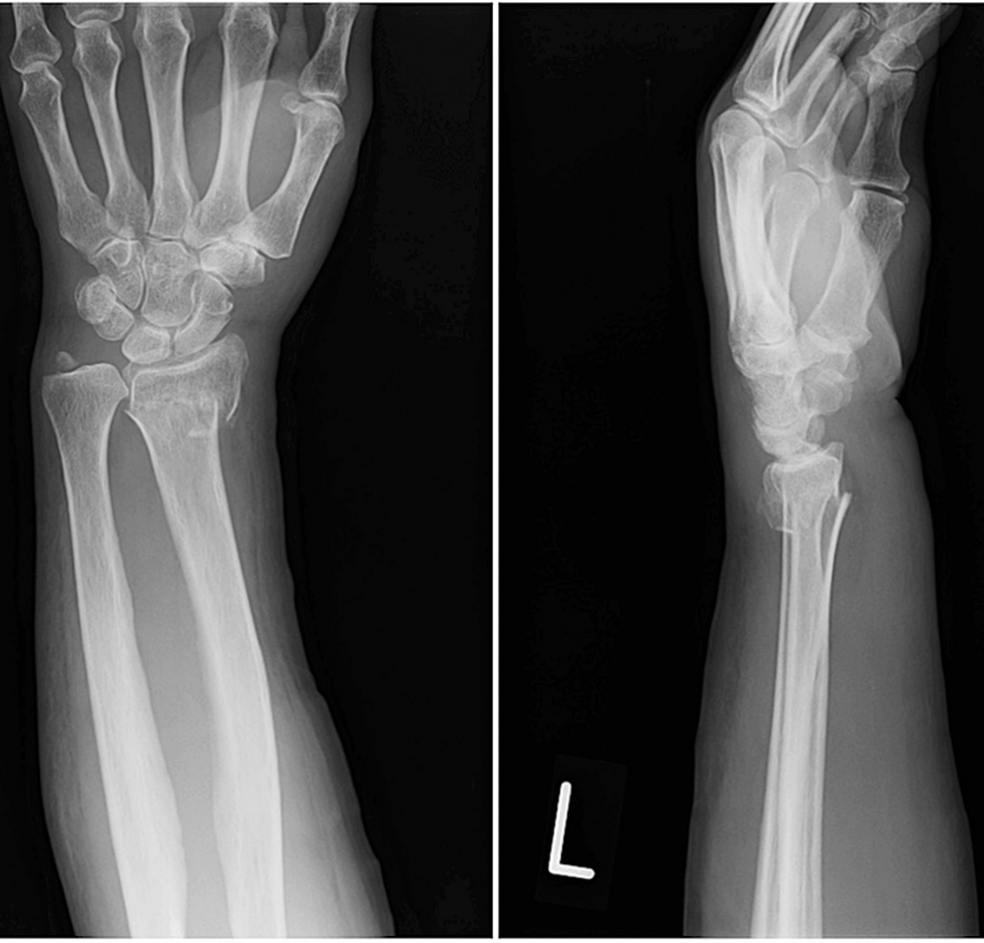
Preoperative posteroanterior and lateral radiographs of the left wrist.
